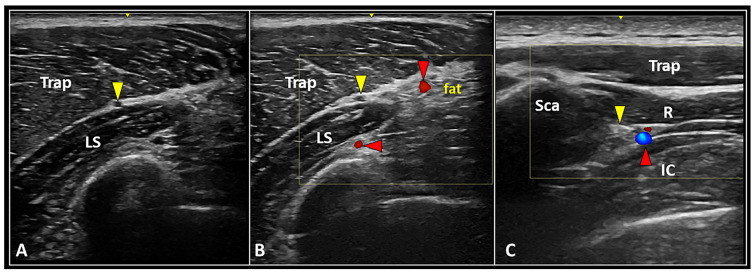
Longitudinal US scan (A) clearly shows the anatomical location of the spinal accessory nerve (yellow arrowhead) within the interfascial space between the trapezius (Trap) and levator scapulae (LS) muscles. Accurately setting the color Doppler box, superficial and deep branches of the transverse cervical artery (red arrowheads) can be promptly identified to plan a safe intervention (B). Transverse ultrasound scan with color Doppler imaging (C) shows the dorsal scapular nerve (yellow arrowhead) and artery (red arrowhead) running inside the interfascial plane between the rhomboid (R) and intercostalis (IC) muscles medial to the medial edge of the scapula (Sca).
